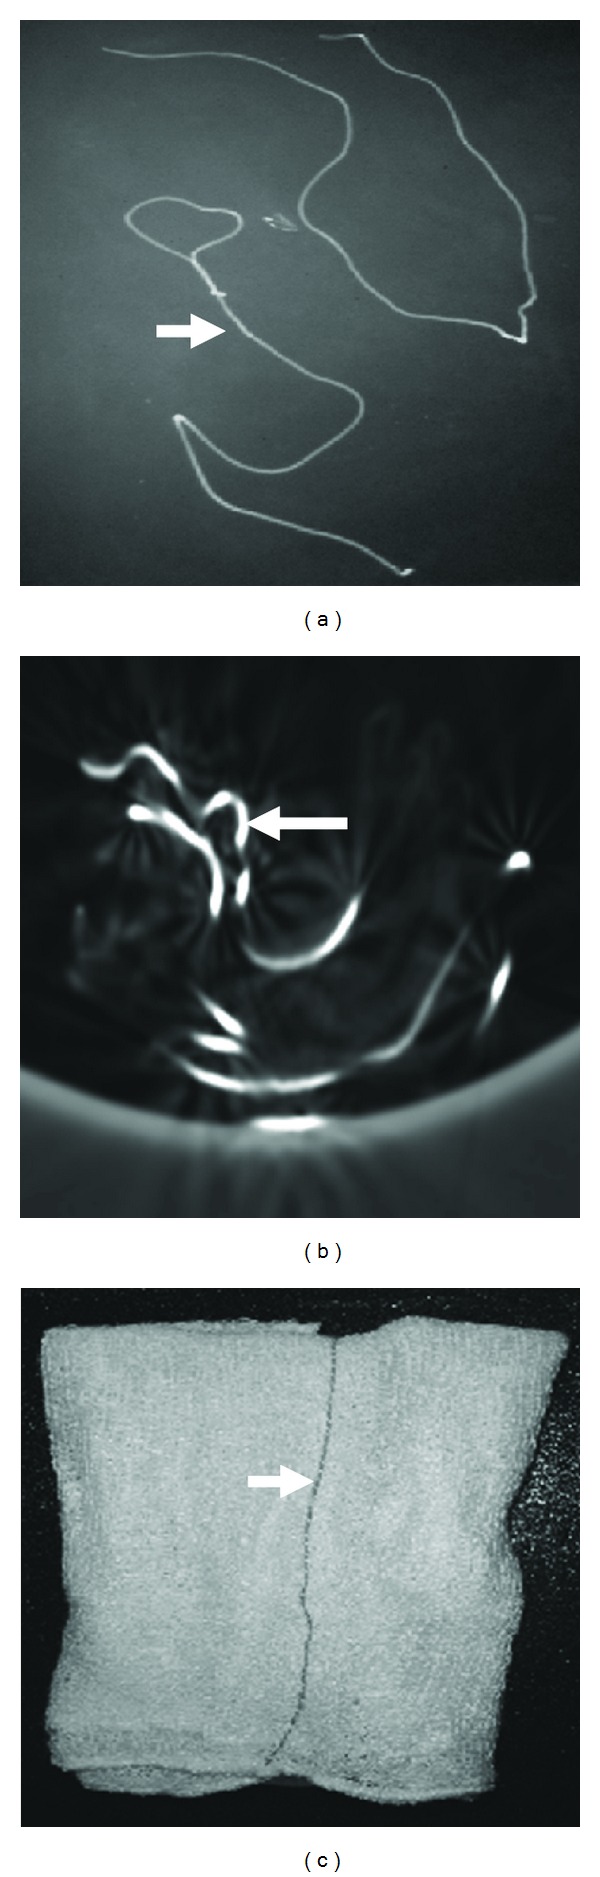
Plain X-ray (a), CT scan (axial section) of the surgical sponge (b), in lung window setting and the photograph of the surgical sponges, having radio-opaque markers (c). Radio-opaque markers are indicated by arrows.
